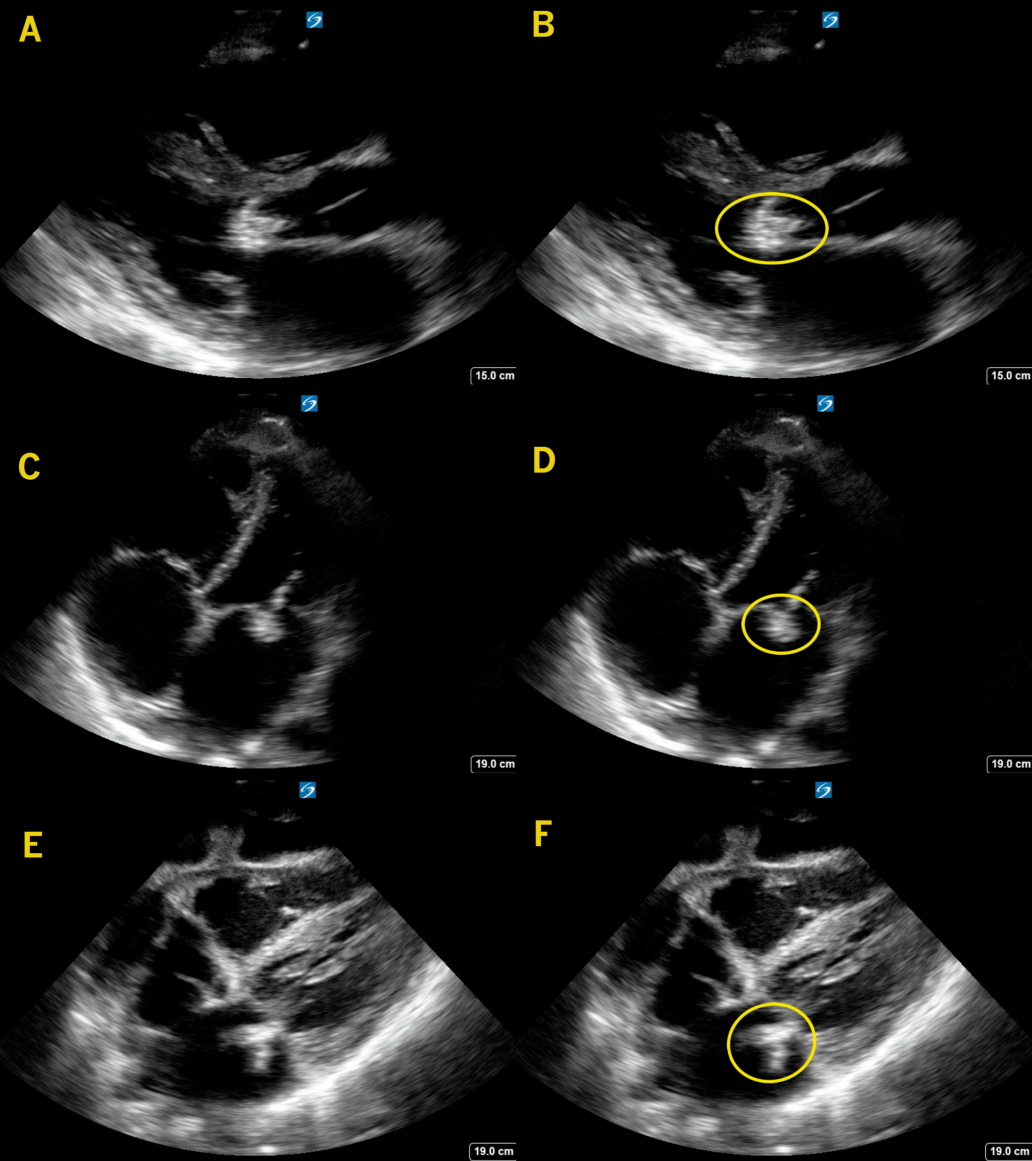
Mitral Valve Vegetation In these images, a large, echogenic mass (circle) is seen attached to the mitral valve from a parasternal long-axis view (A-B), an apical 4-chamber view (C-D), and a subcostal view (E-F). This mass is likely the origin of the embolic arterial disease seen in the axillary artery in image 5.
